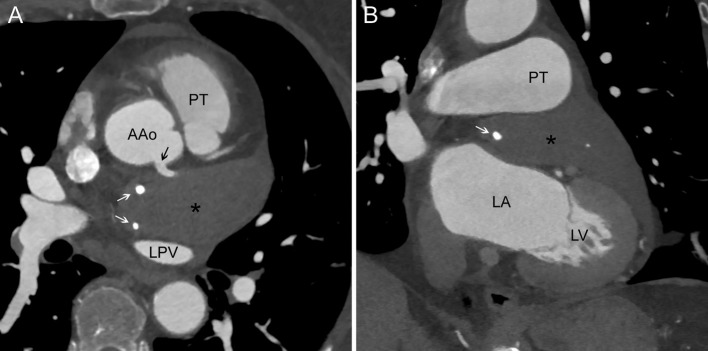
Chest computed tomography. (A) Transverse section. The intrapericardial tumor (asterisk) lies between ascending aorta (AAo), pulmonary trunk (PT), and left pulmonary vein (LPV). It contains two macrocalcifications (white arrows) and is modeled by the left main coronary artery (black arrow). (B) Coronal section. Left atrium (LA) is slightly compressed by the tumor without any signs of invasion. Left ventricle (LV) models inferior part of the tumor, PT models its superior part.
